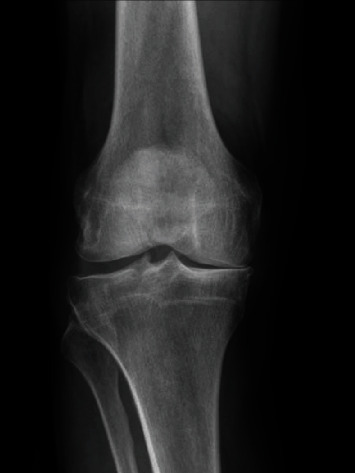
Preoperative radiograph with isolated medial disease.
